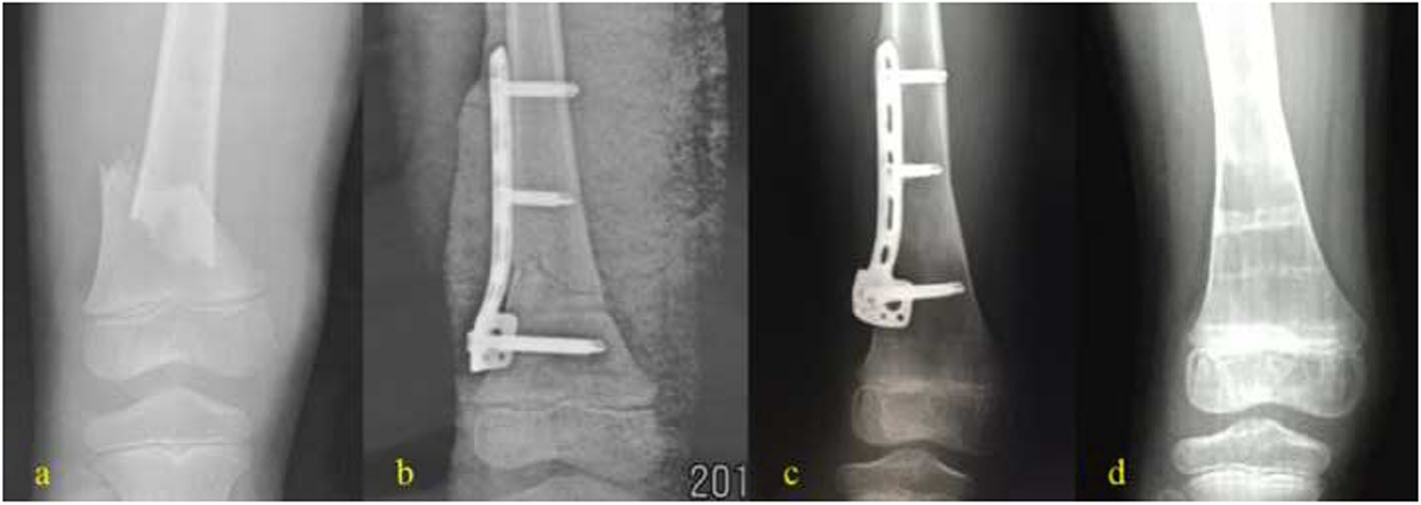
Anteroposterior radiographs showing pediatric displaced supracondylar femoral fractures treated with locking plate. male, 6 years old, right side. a Pre-operation. b Post-operation. c 11 months after surgery. d 18 months after surgery
